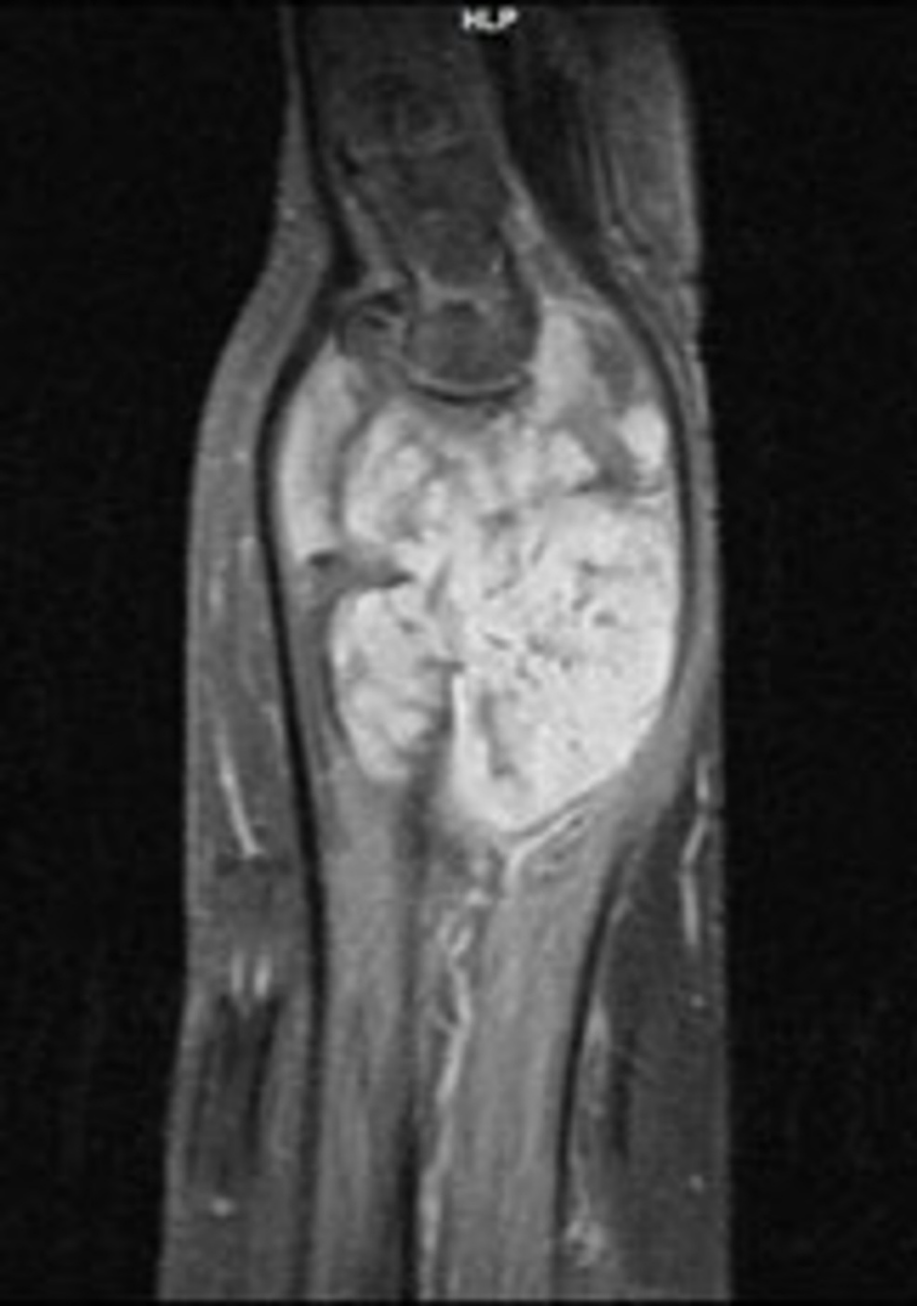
Coronal view of the left wrist (MRI; T2W image). The lesion shows the characteristic “target pattern.” MRI: magnetic resonance imaging; T2W: T2-weighted
